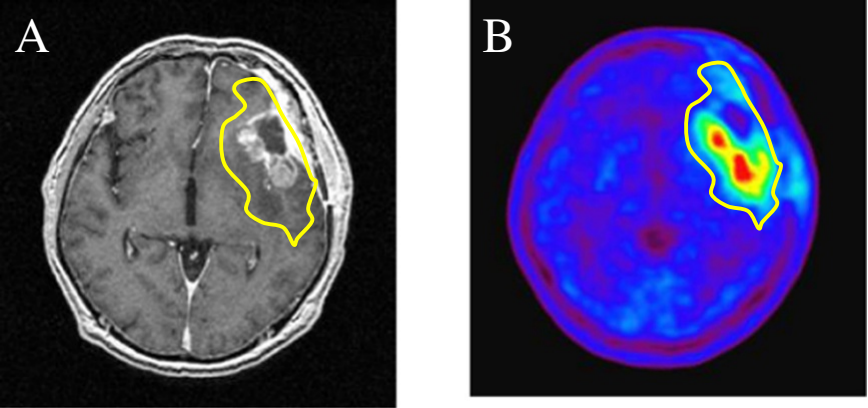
An example of a target planned for a hypofractionated stereotactic radiotherapy using intensity modulated radiation therapy. (A) Contrast-enhanced T1-weighted magnetic resonance imaging. (B)
